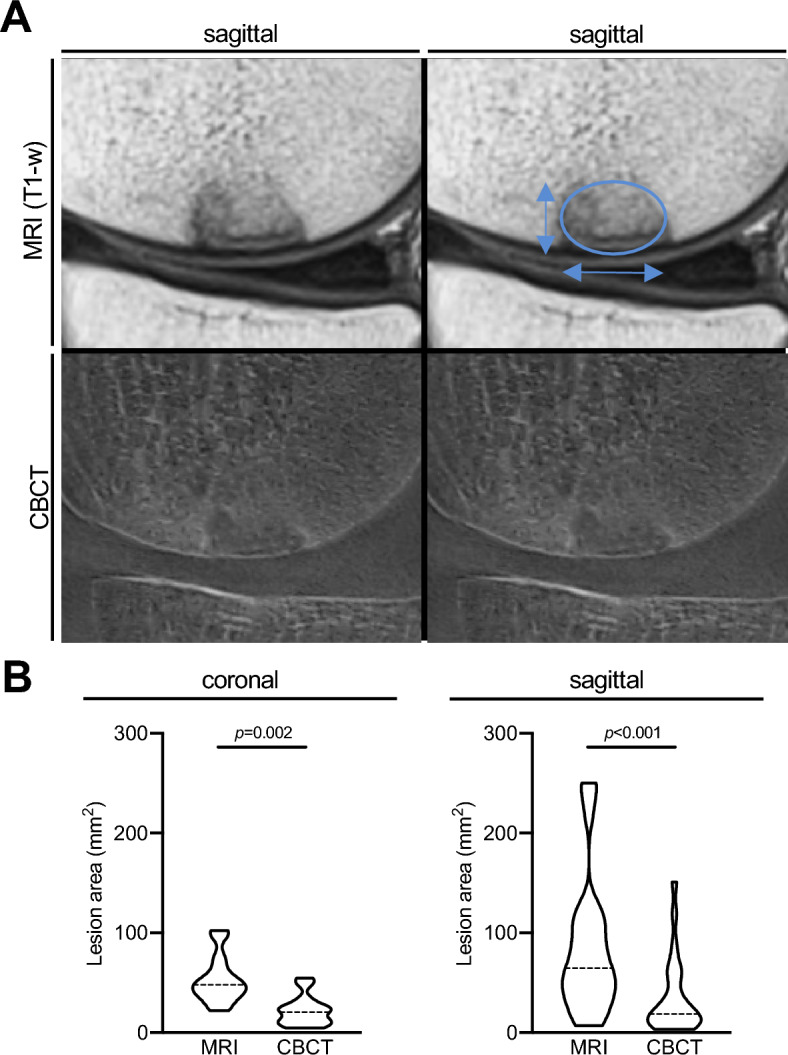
Measurement of the osseous lesion size using an elliptical area between MRI and CBCT. (A) Exemplary MRI (T1) and CBCT scans in sagittal orientation of a patient’s medial femoral condyle with measurement of the bony lesion. (B) Comparison of bony lesion size measured in coronal and sagittal views between MRI and CBCT. *MRI* magnetic resonance imaging, *CBCT* cone beam computed tomography, *T1-w* T1-weighted.
